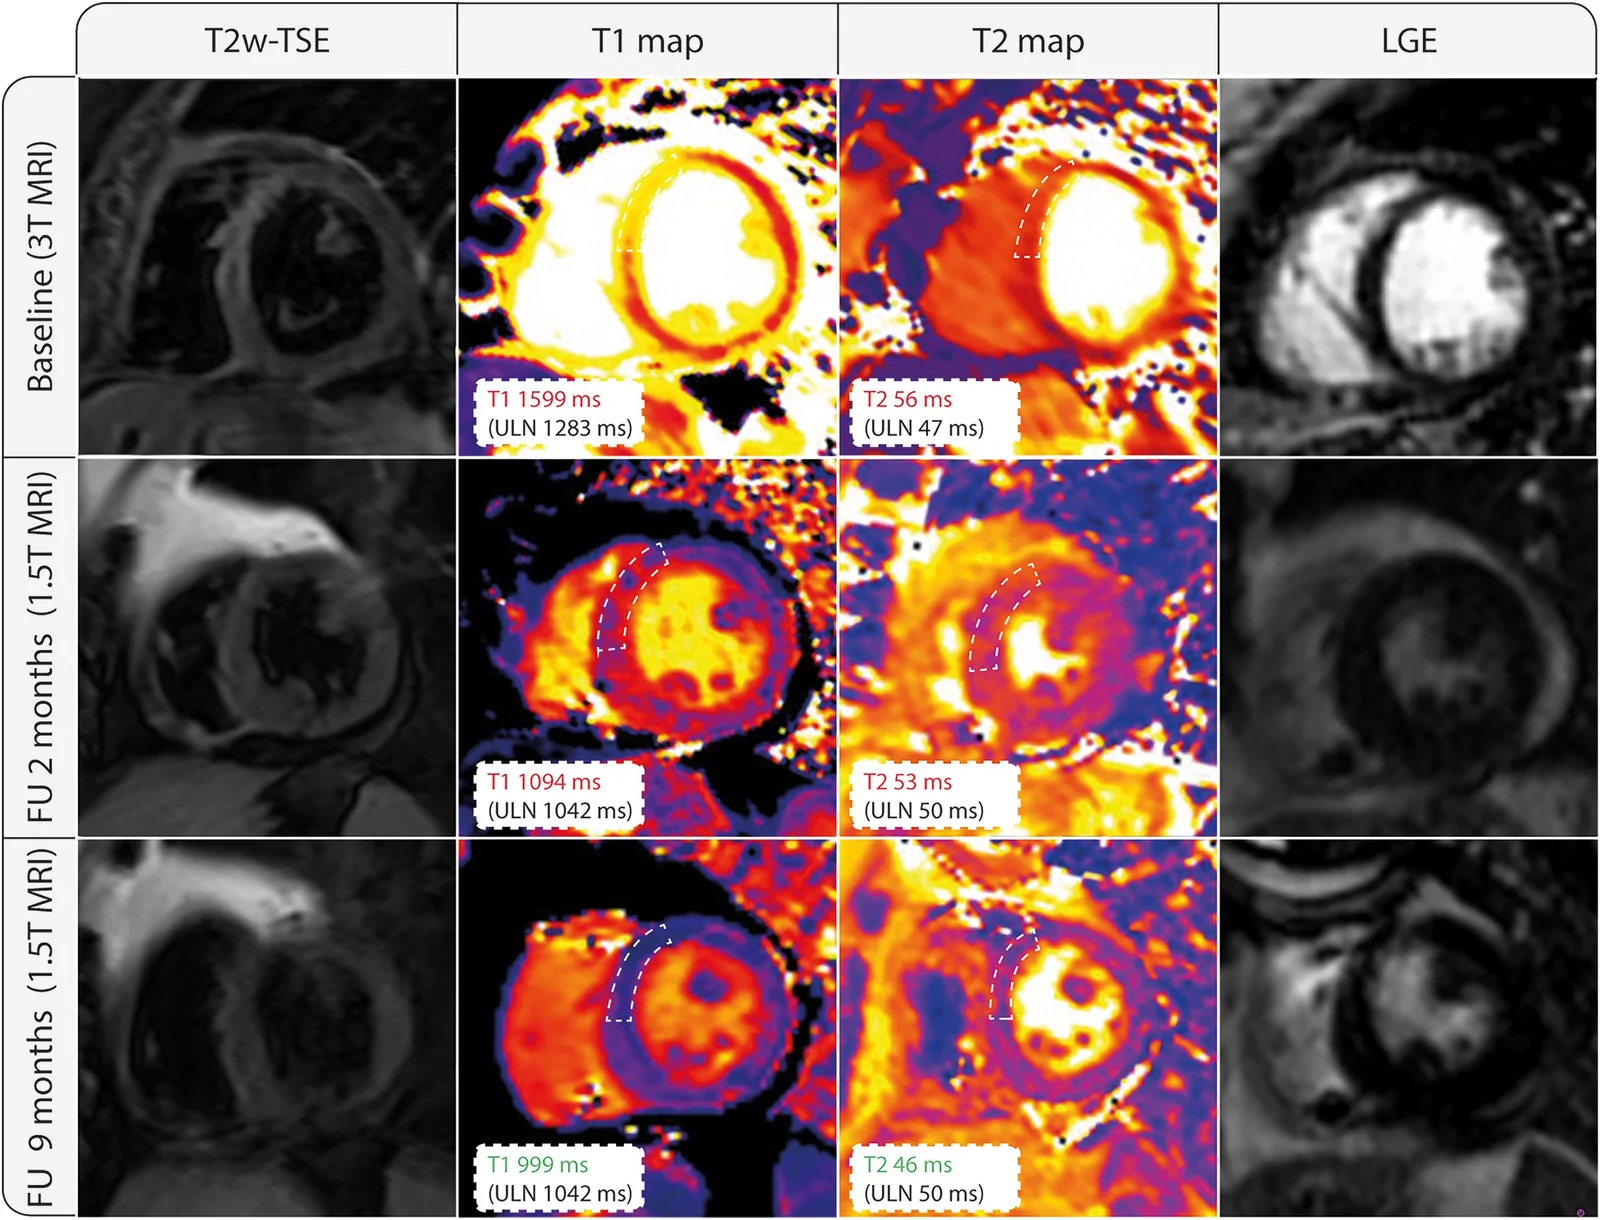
CMR findings in Case 1, a patient with SLE myocarditis. Baseline CMR demonstrated increased signal intensity basal anteroseptal on T2-weighted turbo spin echo (T2w-TSE), globally increased myocardial T1 and T2 values on mapping, anteroseptal mid, respectively, 1599 and 56 ms, and no late gadolinium enhancement (LGE). CMR imaging after 2 months showed persisting albeit decreased myocardial inflammation with normal T2w-TSE images but slightly elevated T1 and T2 values on mapping (1094 and 53 ms anteroseptal mid, respectively) and no LGE. CMR imaging after 9 months demonstrated complete normalization without signs of myocardial inflammation on T2w-TSE and T1 and T2 mapping and absence of LGE. Abbreviations as in *Figure 1*.
